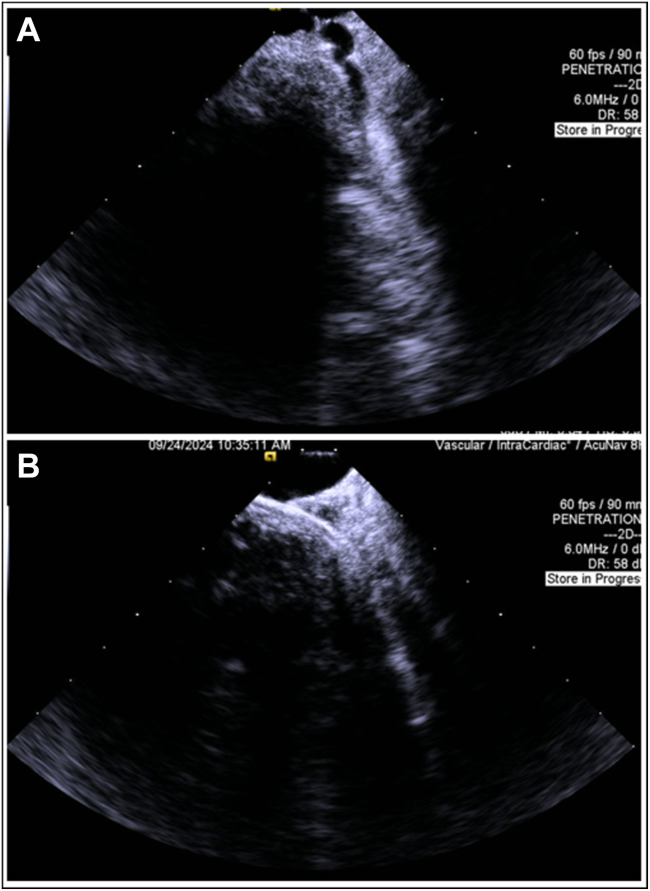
A–B: Intracardiac echocardiography visualization of the coronary sinus (CS) anatomy and successful cannulation of a lateral CS branch using an extended hook sheath.
